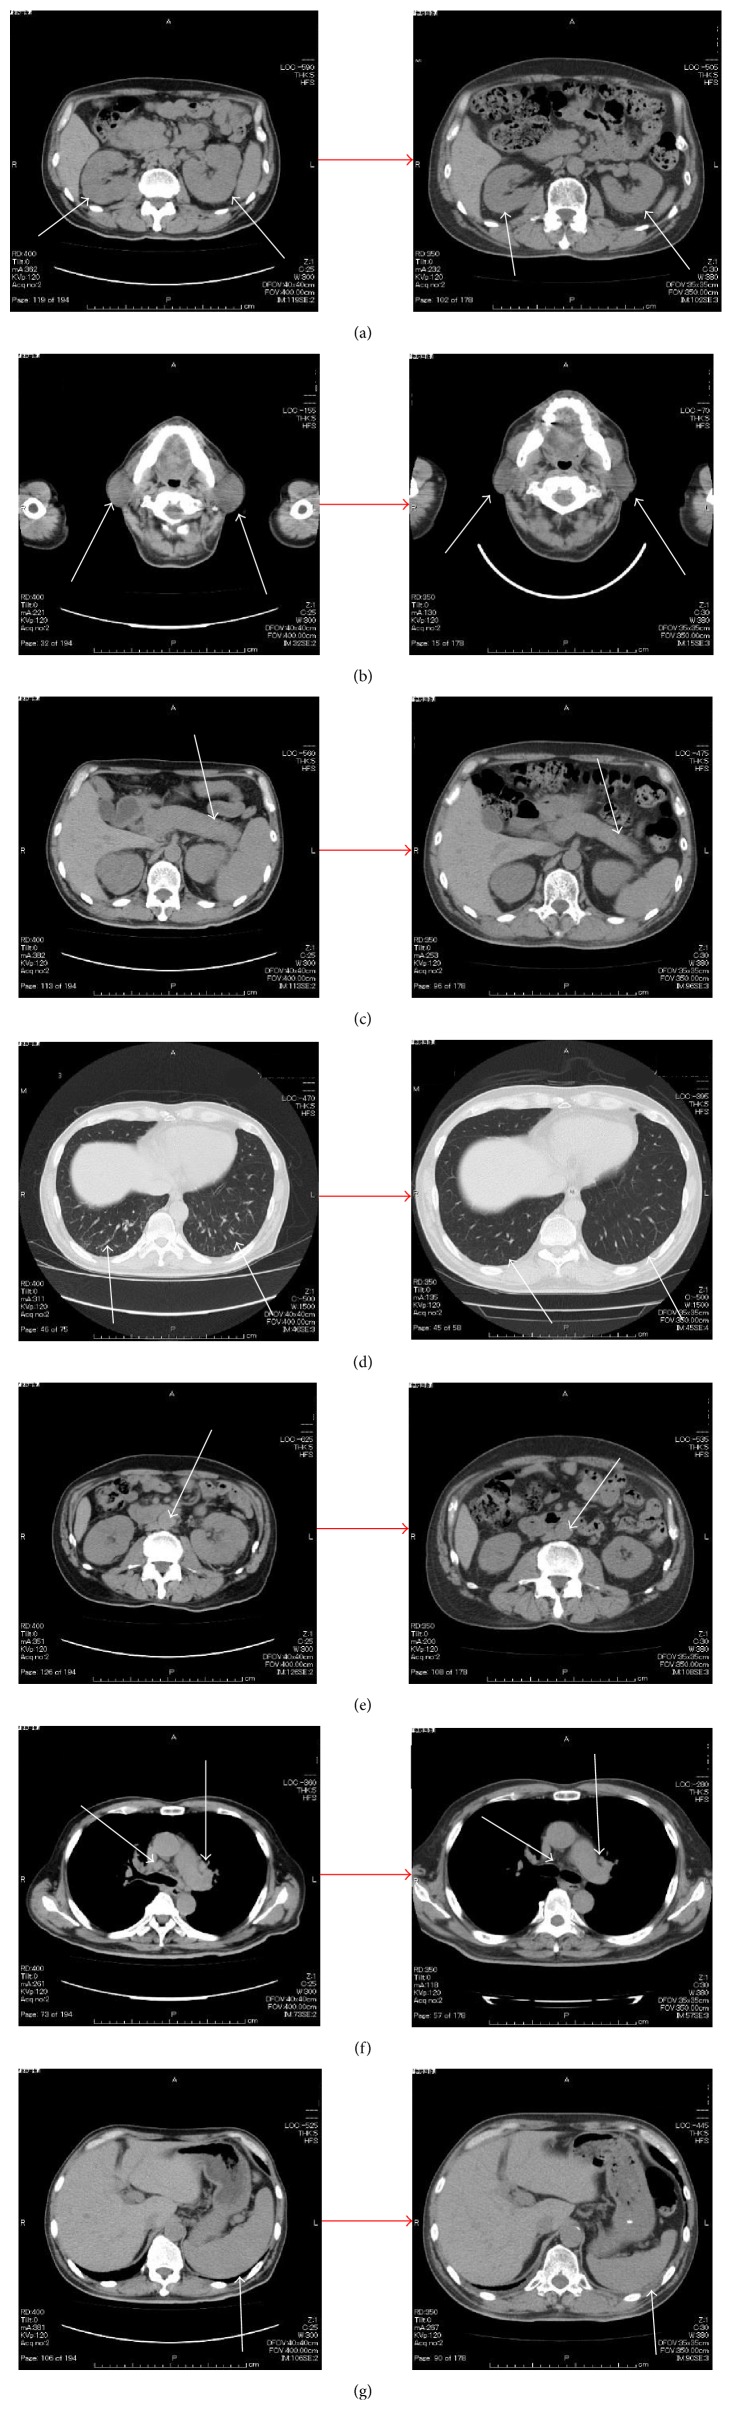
Computed tomography. It showed the improvement of renal enlargement ((a), white arrows), swelling of bilateral salivary glands ((b), white arrows), enlargement of the pancreas ((c), white arrows), centrilobular shadows ((d), white arrows), soft tissue shadows around the aorta ((e), white arrows), mediastinal and pulmonary lymph nodes ((f), white arrows), and splenomegaly ((g), white arrows).
